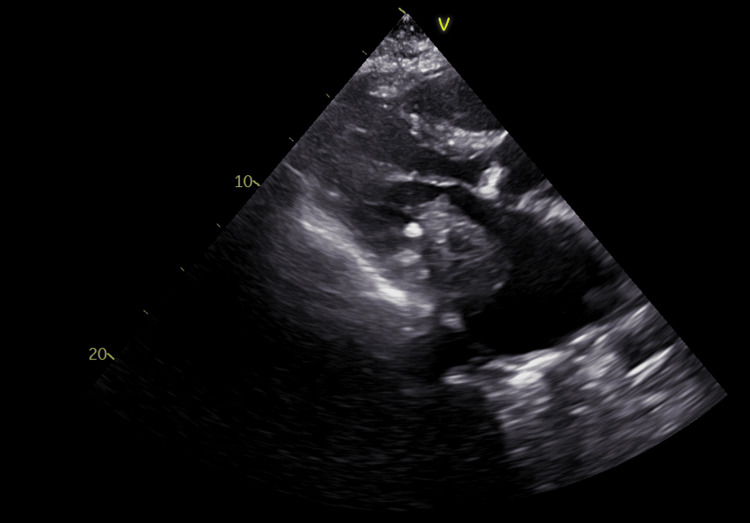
Transthoracic echocardiogram showing the parasternal long-axis view
